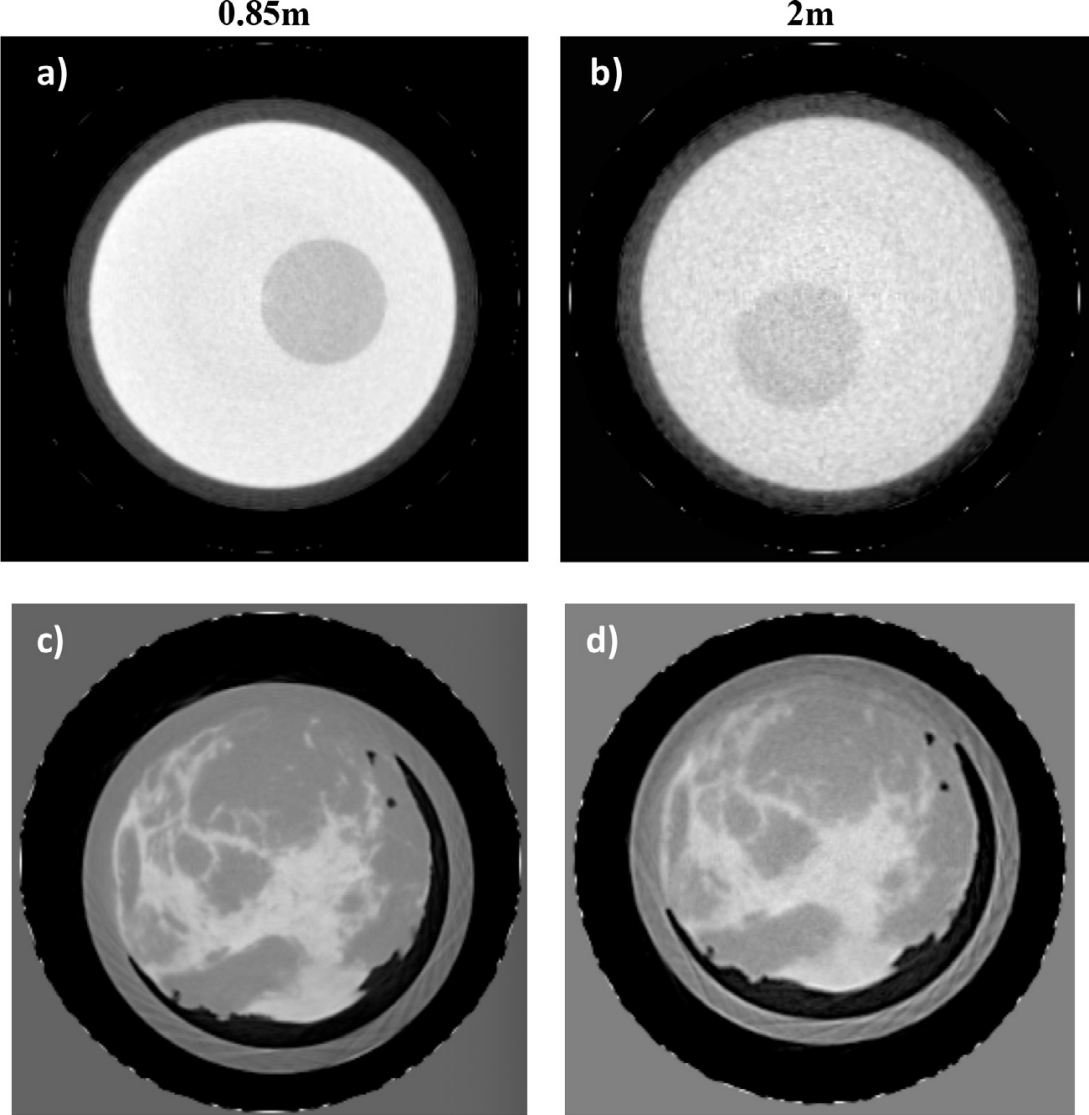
Computed Tomography (CT) transverse slices reconstructed from single shot
